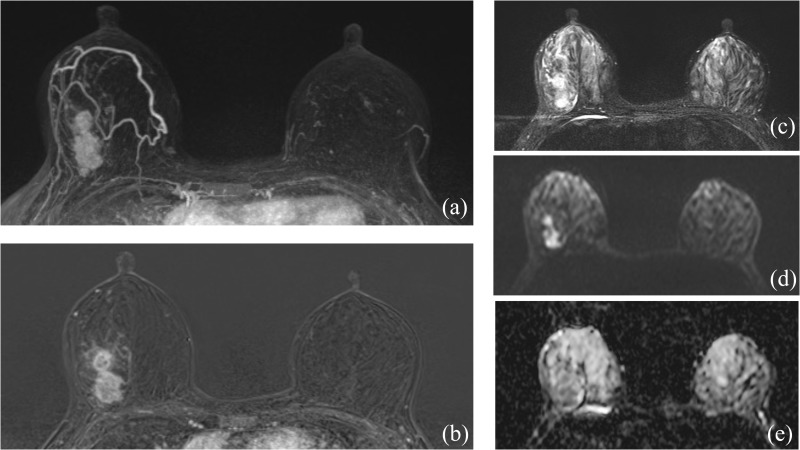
A 59-year-old female presented with a palpable mass in her right breast. After undergoing breast conserving surgery, the final pathology report revealed a grade III, ER-negative, PR-negative, and Her-2 positive squamous carcinoma. There were no axillary lymph node metastases identified. (1a-b) Breast MRI showed a dumbbell-shaped, heterogeneously enhancing mass with an irregular shape and margin, located in the upper outer quadrant of right breast. (1c) T2-weighted image showed iso- to hyper signal intensity of the tumor. (1d-e) the diffusion-weighted image showed hyperintensity and the corresponding ADC value showed 1.49x10^-3^ mm^2^/s.
